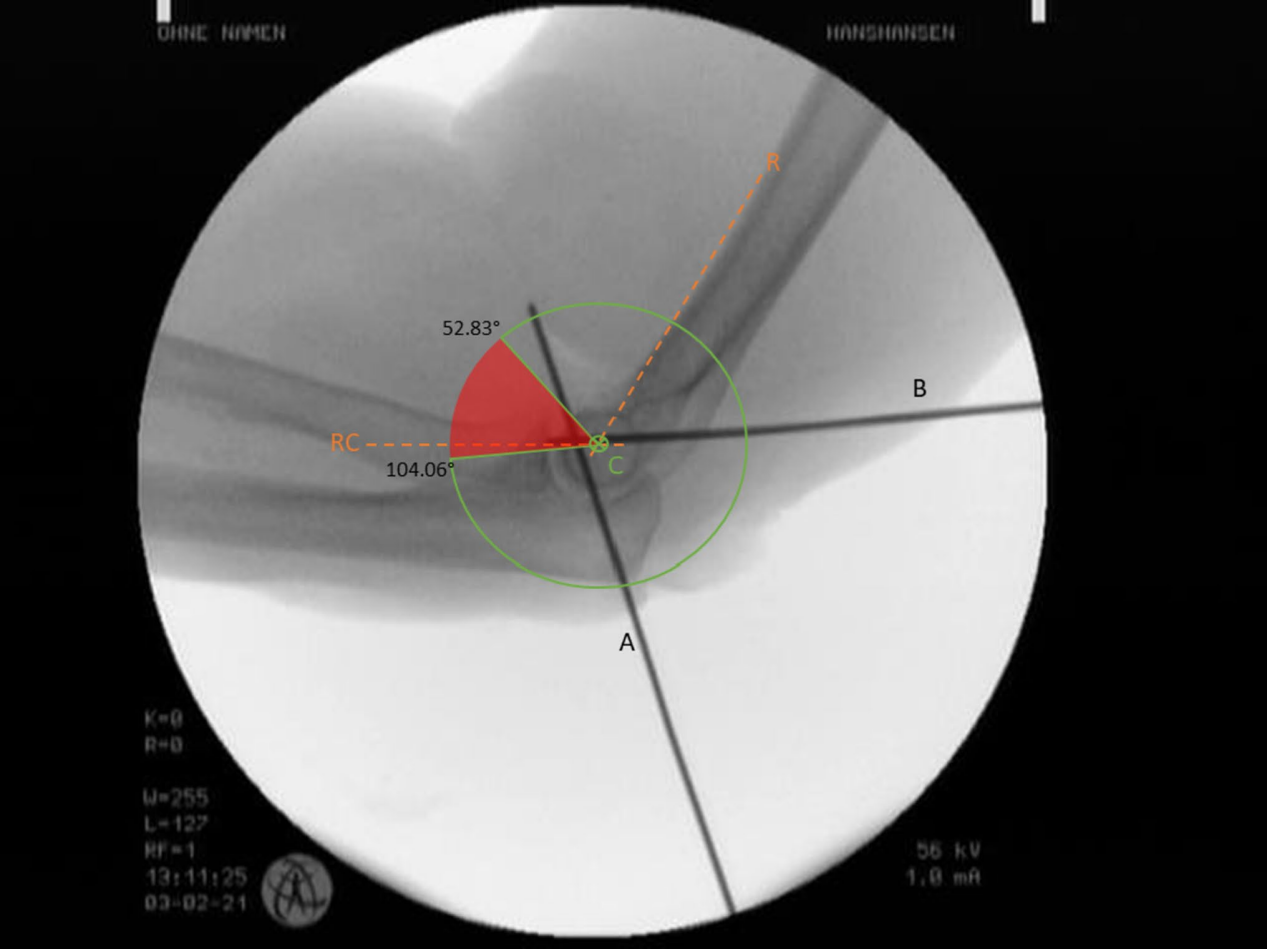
Lateral radiograph with the rogers line (R) and the radio capitellar line (RC) intersecting in the center of rotation (C). The intersection of the capitellar surface with both K-wires (A and B) is marked and the angle between R and the intersection is digitally measured, leaving the corridor which is not reachable (red surface) in between
